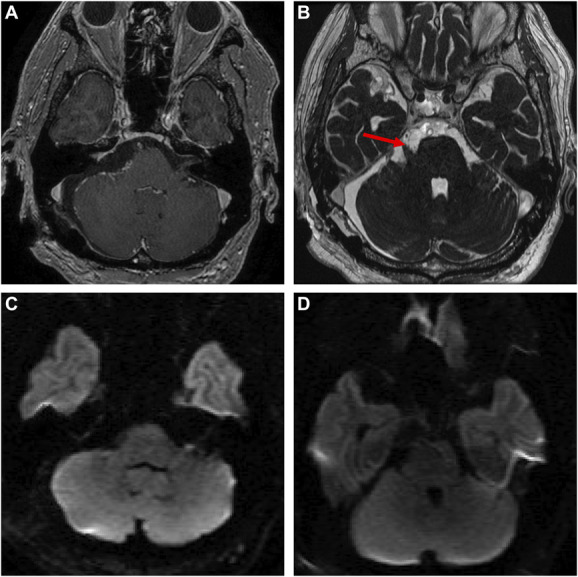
Postoperative magnetic resonance images. A, Gadolinium-enhanced T1-weighted imaging showed near-complete tumor resection and relief of brainstem compression. B, The trigeminal nerve was clearly visualized on constructive interference in steady state imaging (*arrow*). C, and D, Diffusion-weighted imaging showed no ischemic changes in the cerebellum or brainstem.
